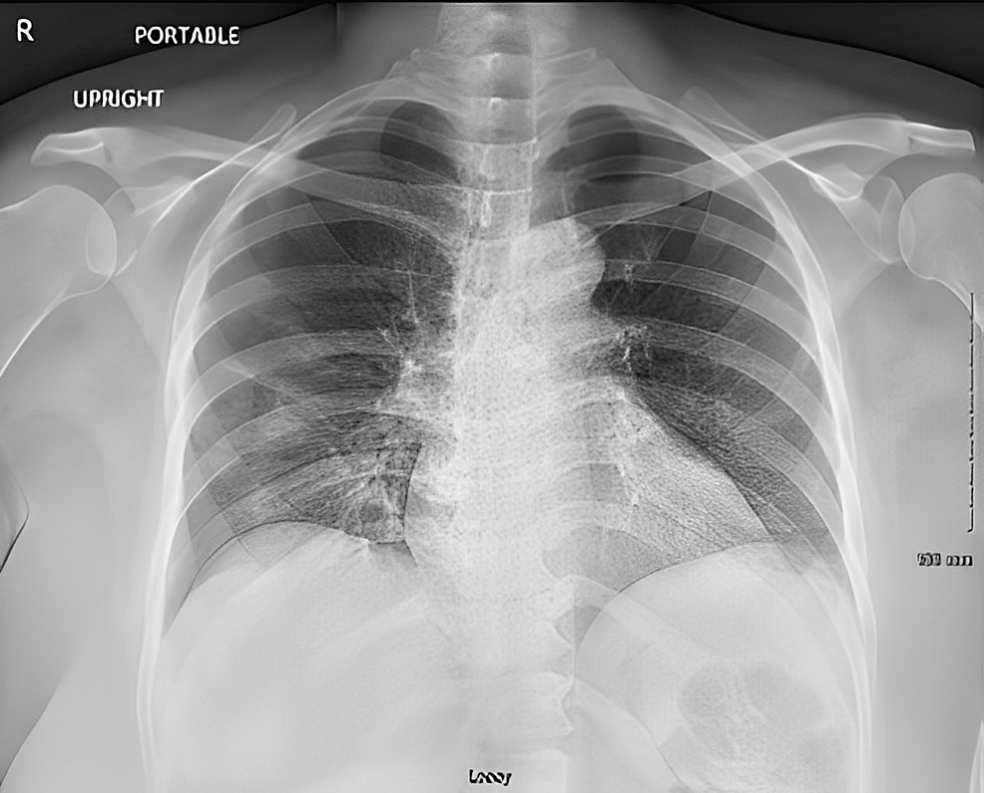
Image of chest x-ray on admission showing interstitial opacities in lung bases.
